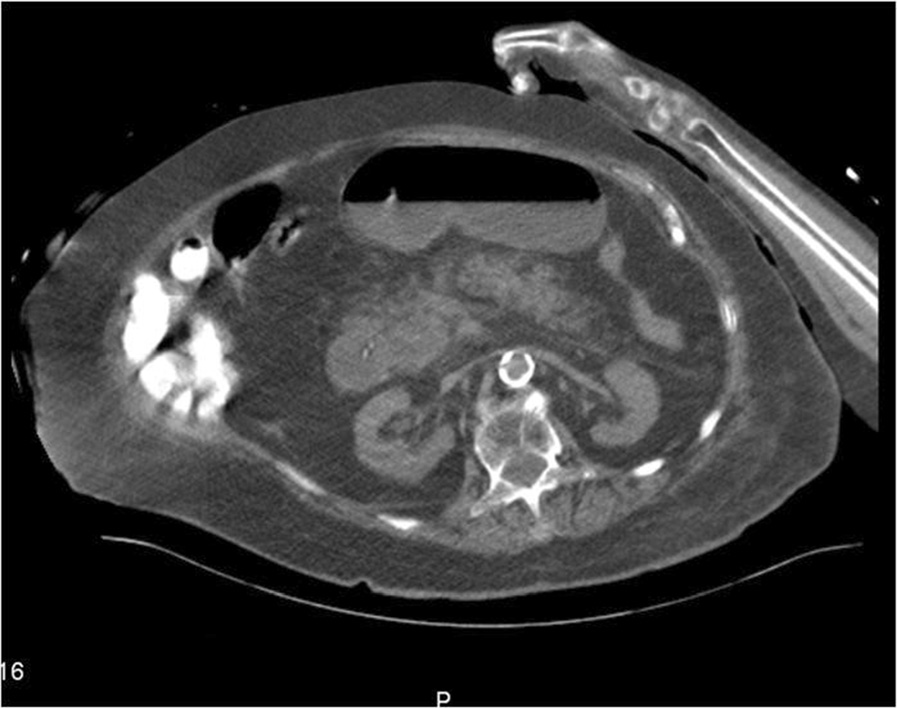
Abdominal CT scan showing Foley catheter balloon located in the second part of the duodenum and peripancreatic fat stranding with an edematous pancreatic head.
